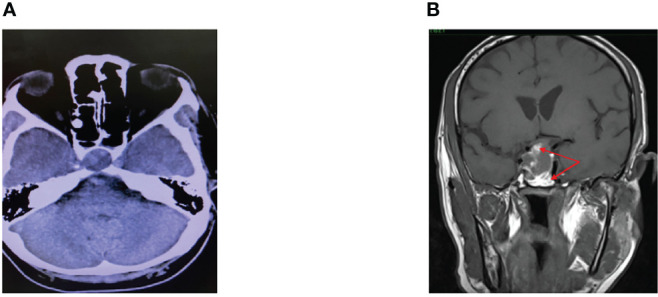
(A) CT scan of the brain. (B) MRI pituitary T1 coronal section.
